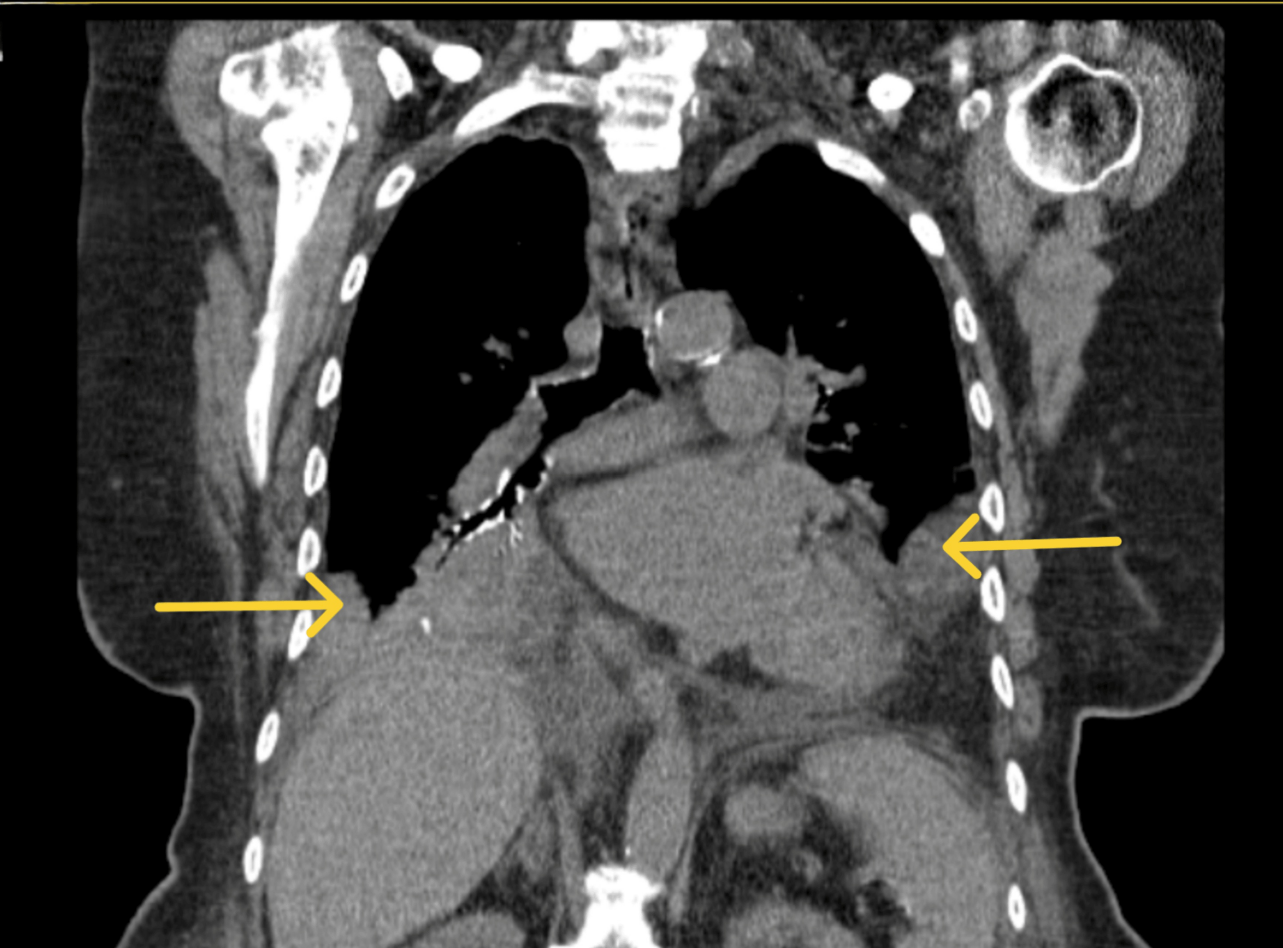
CT chest showing bilateral pleural effusions (R > L) with adjacent RLL possibly consistent with pneumonic process and likely parapneumonic effusion, correlate clinically Yellow arrows show signs of parapneumonic effusion. RLL: right lower limb; R: right; L: left
